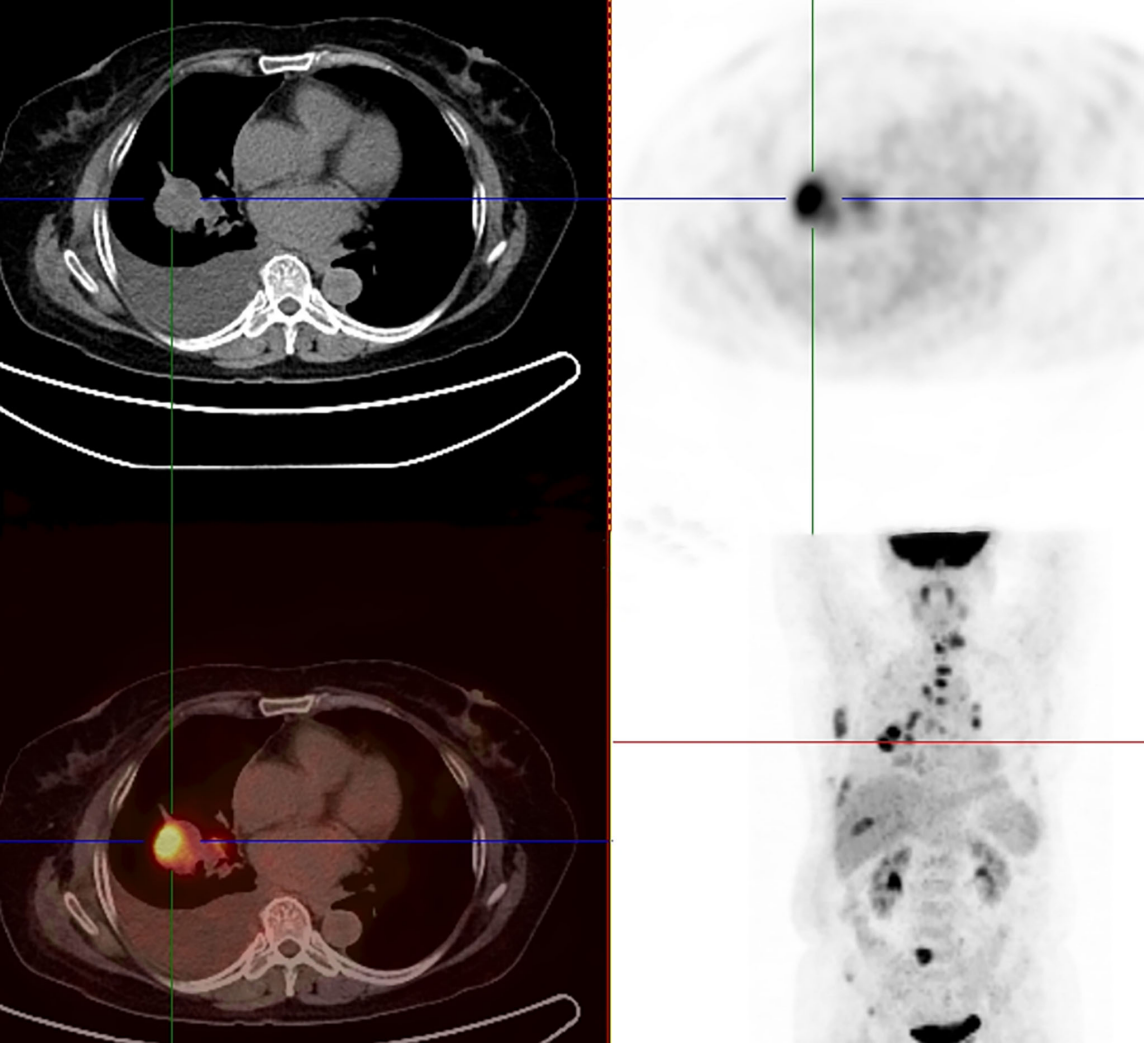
^18^F-FDG-PET/CT showed a right lower lobe malignant tumor (4.2 cm×3.3 cm×4.8 cm, SUVmax 9.6) with multiple right lung metastases and metastatic right pleural effusion.
